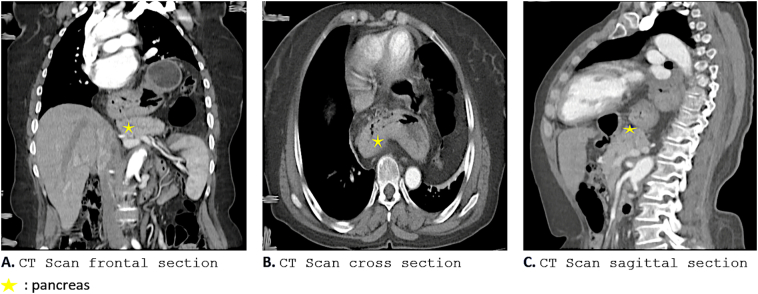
CT scan views showing the Intrathoracic position of the pancreas (yellow asterisk). (For interpretation of the references to colour in this figure legend, the reader is referred to the web version of this article.)
